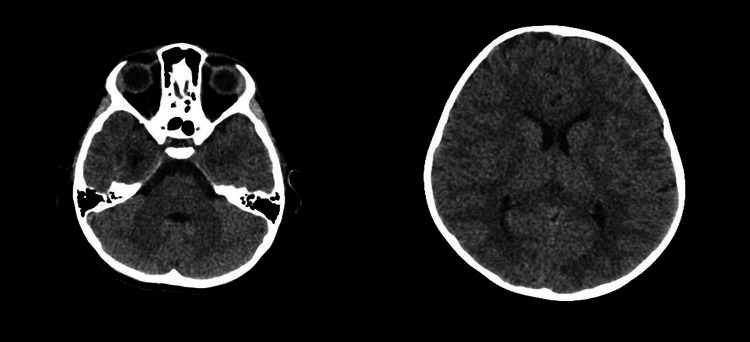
The CT of the brain is revealed to be normal (no evidence of intracranial bleeding or intracranial hypertension)
